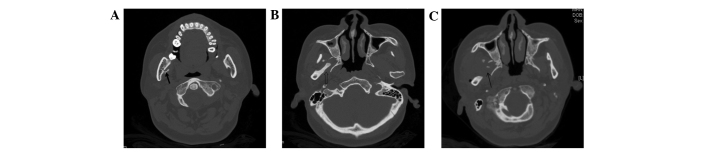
(A) Preoperative computed tomography (CT) scan demonstrating calcification in the right medial pterygoid muscle (solid arrow). (B) Preoperative axial CT showing calcification in the right lateral pterygoid muscle (arrow outline); (C) Postoperative CT scan demonstrating that the ossification was excised (arrow).
